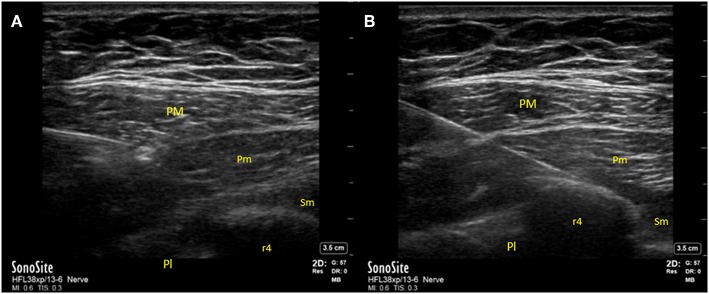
Ultrasound image with identification of relevant structures for Pectoralis plane block. (A) PECS I block and (B) PECS II block. PM, pectoralis major muscle; Pm, pectoralis minor muscle; Sm, Serratus anterior muscle; r4, fourth rib; Pl, pleura.
